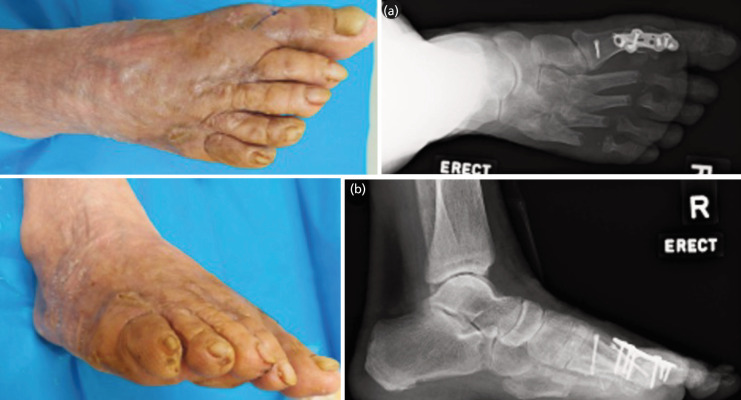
Latest clinical photographs of the right foot, as well as (a) AP and (b) lateral weight-bearing radiographs at the three-month follow-up.
